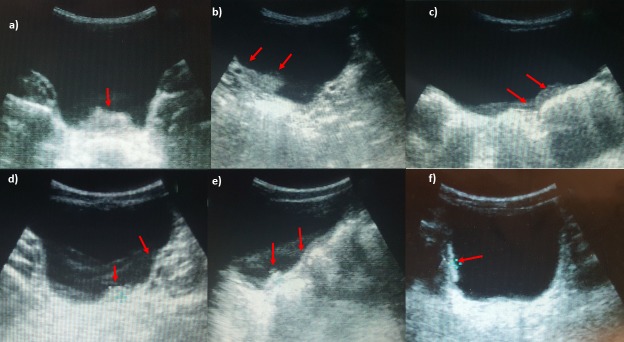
Ultrasonography images of urinary bladder of PSAC infected with *S*. *haematobium*. a) focal thickening of the bladder wall, longitudinal plane shows mass-like lesion (arrows); b) transverse plane image shows diffuse thickening of the bladder wall more evident in the right posterior wall; c) transverse plane image shows a focal heterogeneous echo of the bladder wall in absence of true thickening or mass-like lesions in the lumen; d) longitudinal plane shows a marked and diffuse thickening of the bladder left wall with a mass like lesion (arrow); e) image of diffuse and marked thickening of the bladder wall with pseudo-polyp lesion; f) focal thickening of the wall evident on the right wall and diffuse heterogeneity of bladder echo.
